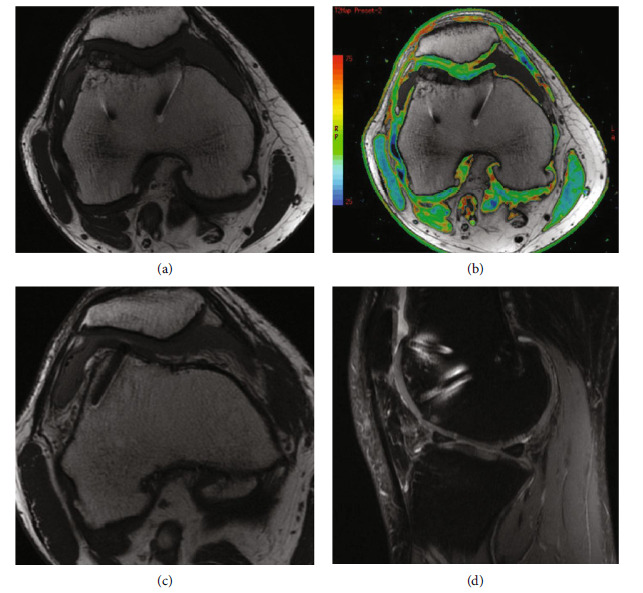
Two-year postoperative MRI. (a) Axial metal artifact reduction sequence (MARS) image demonstrates previous trochlear osteochondral allograft well integrated with intact articular cartilage. (b) T2 mapping axial image with thinning of medial and lateral patellar facets. (c) Additional axial image. (d) Sagittal MARS proton density-fat suppression image demonstrating maintained trochlear implant congruity and bone incorporation; slight thinning at the inferior patellar articular cartilage is demonstrated as well.
